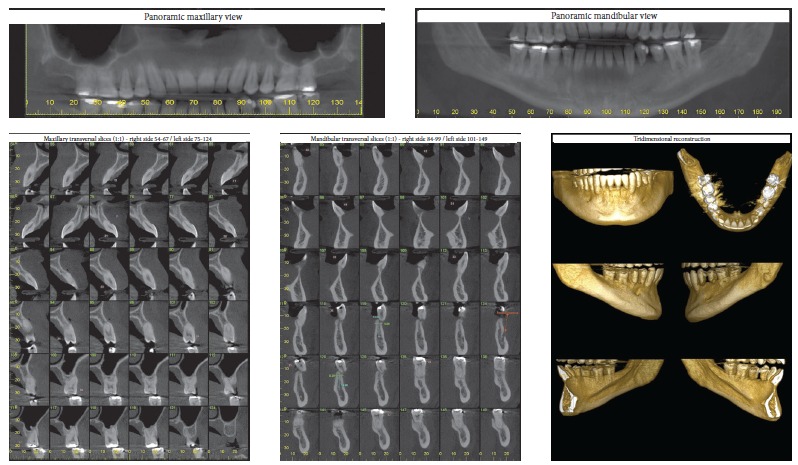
Baseline cone-beam CT scan.
